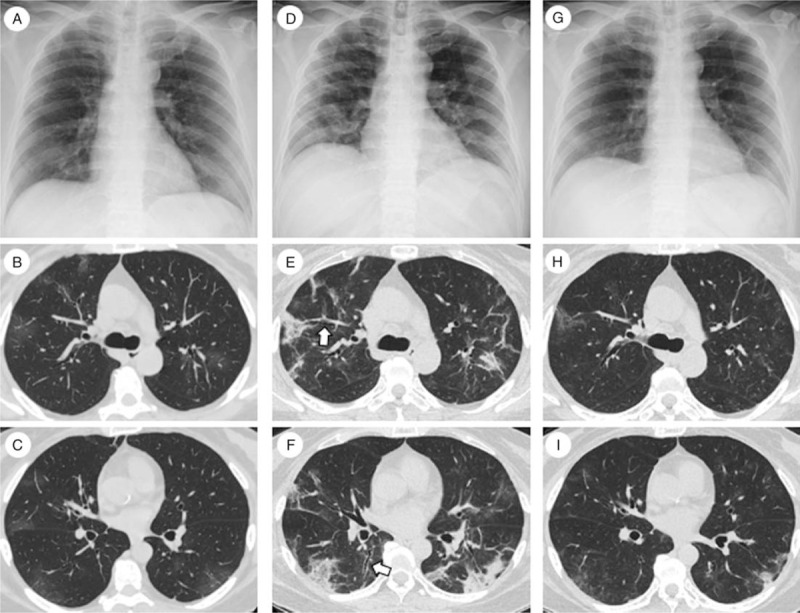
Chest imaging of Case 2. (A) Chest X-ray on admission. A slight ground-glass opacity (GGO) was observed in the right lower lung field. (B, C) Chest computed tomography (CT) on admission showed bilateral faint GGO. (D) Chest X-ray on day 6 post hospital admission (immediately before corticosteroid administration) showed bilateral GGO. Lung volume was markedly decreased. E, F: Chest CT on day 6. Consolidations developed bilaterally, some of which producing band-like shapes, and were distributed in subpleural or peri-bronchial regions. Traction bronchiectasis was observed (white arrow). A (G) Chest X-ray and (H, I) chest CT on day 17 revealed improvement of lung infiltrates and the recovery of lung volume. CT = computed tomography.
